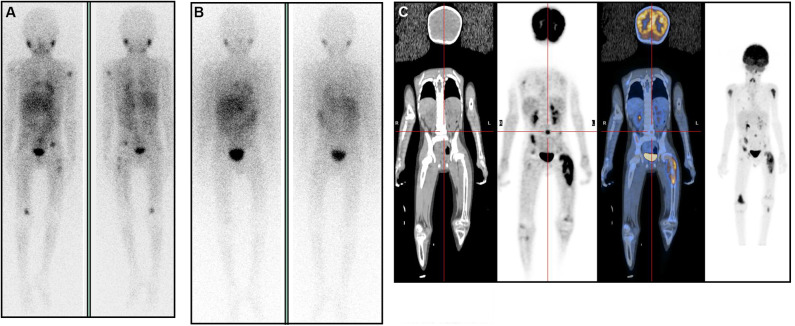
False complete response after 131-I-mIBG therapy: (A,B) 123-I-mIBG scans. (C) 18-F-FDG-PET-CT scan. (A) positive scan previous to 131-I-mIBG therapy, with multiple spots. (B) negative post-mIBG therapy scan (Complete Response). (C) as response was much better than expected, a post-therapy PET-CT scan was requested, showing non-mIBG-avid Progressive Disease.
